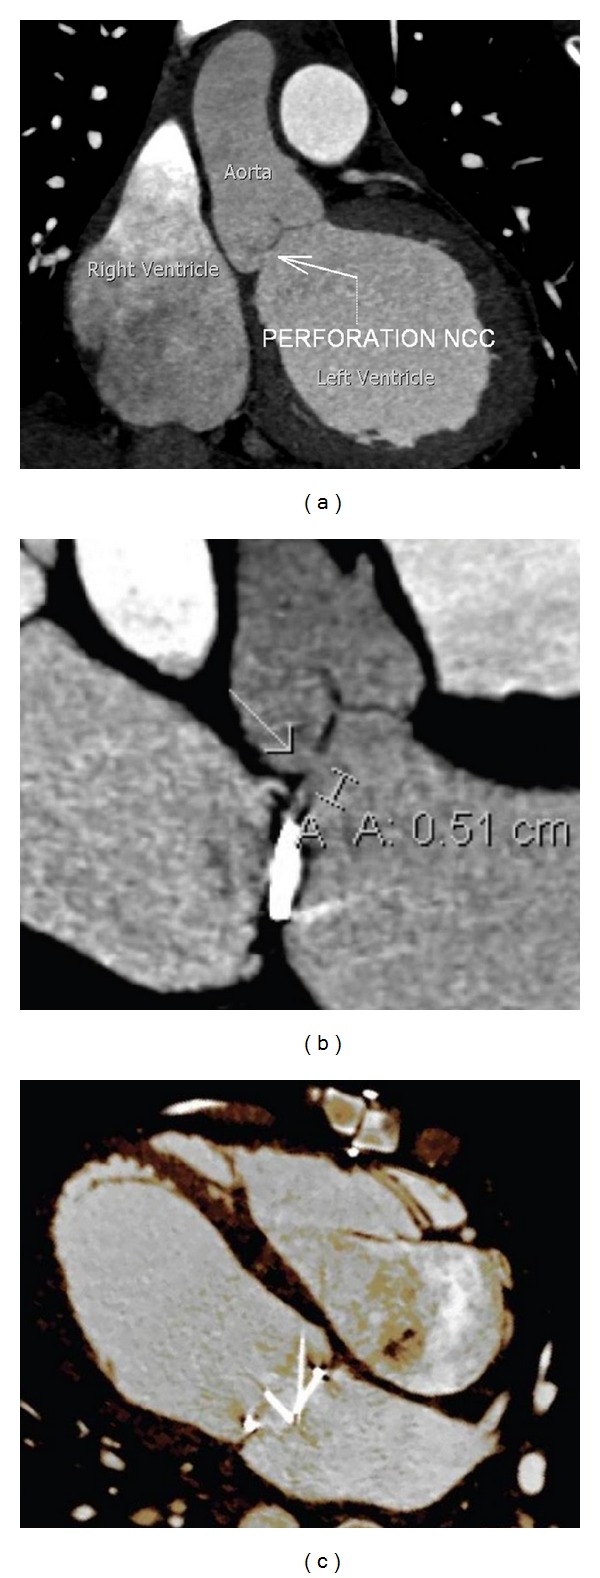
Cardiac gated computed tomography. (a) Large and (b) magnified oblique reformatted images of the left ventricular outflow tract and aortic valve demonstrate a clearly visible 5 mm defect in the non-coronary cusp of the aortic valve. (c) Four-chamber orientation shows the asymmetric movement of the bileaflet mechanical mitral valve due to the regurgitant jet originating from the aortic valve defect. Supplementary CINE file online shows 3D rendering of paradoxical mitral valve motion (see supplementary CINE file).
